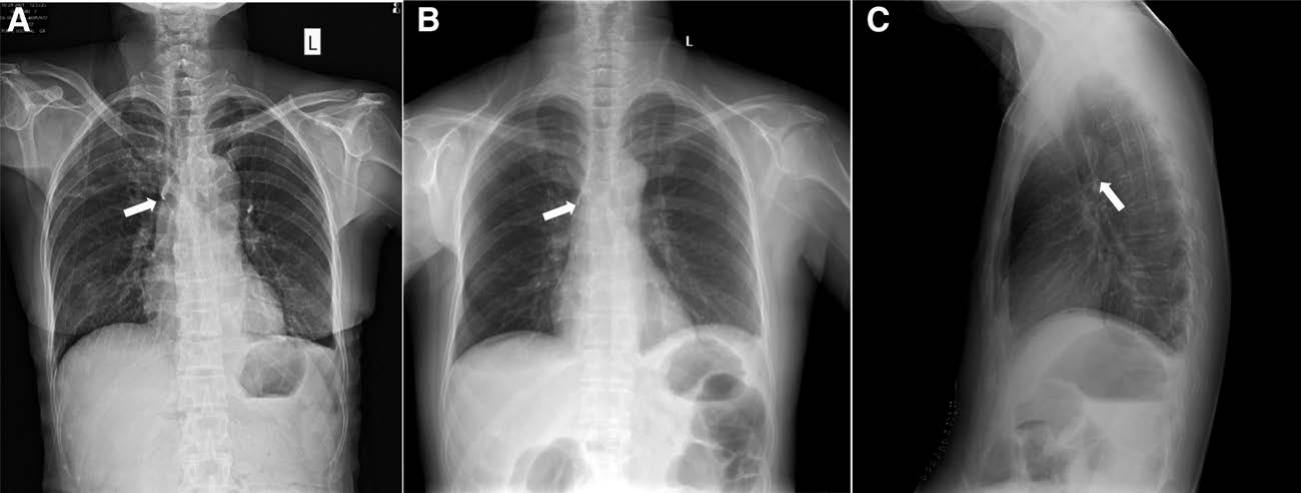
(A, B) Posterior/anterior chest X-ray films showed that the catheters of 2 patients were folded back (white arrows). (A) for patient A, (B) for patient B, and (C) The lateral chest X-ray of patient B clearly showed that the catheter was folded back and entered the azygos vein.
